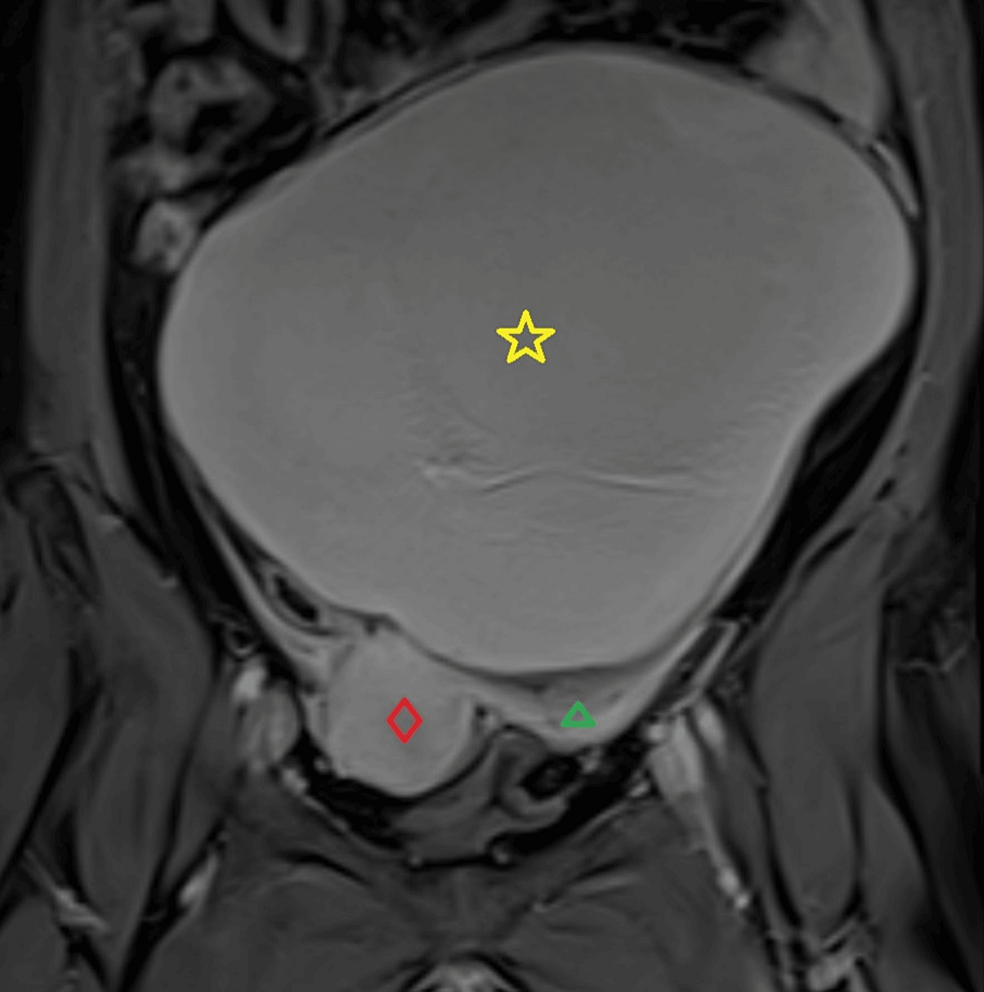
Preoperative MRI showing the left ovarian endometrioma (yellow star) with no features suspicious for malignancy, measuring 20.2 cm in maximal diameter, compressing the uterus (red rhombus) to the patient’s right side, and also showing the left hydrosalpinx (green triangle).
